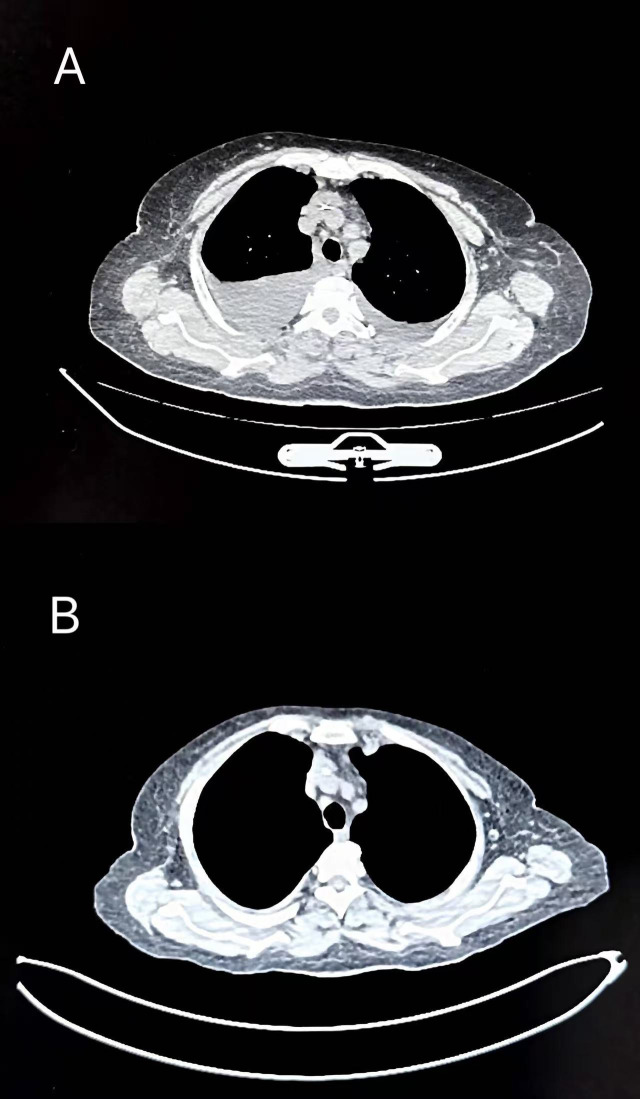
Chest CT findings. (A) Massive bilateral pleural effusion at diagnosis. (B) Near-complete resolution of pleural effusion (Chest CT performed on Day 32).
